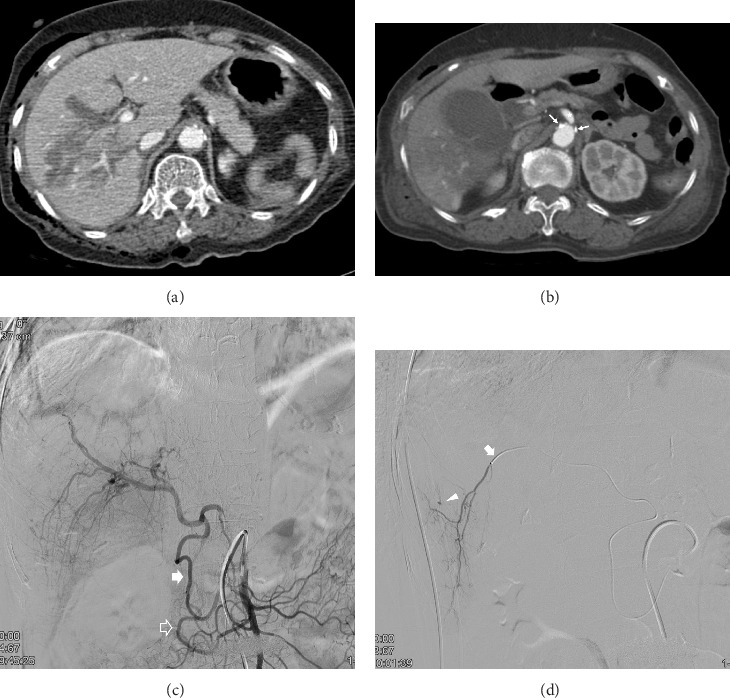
A 77-year-old female presented with blunt hepatic injury caused by a pedestrian traffic incident. (a) Contrast-enhanced (CE) axial CT during the portal phase demonstrates an irregular low-attenuation area suggestive of hepatic parenchymal laceration in the right lobe of the liver. (b) CE axial CT during the arterial phase indicates celiac axis stenosis (CAS) (represented by asterisk) with calcified plaques (arrows) at its origin. (c) Following unsuccessful attempts to cannulate the celiac axis (not depicted), superior mesenteric angiography reveals retrograde contrast material filling of the hepatic artery via the anterior (solid arrow) and posterior (open arrow) pancreaticoduodenal arcades, which originate separately from the superior mesenteric artery. (d) The microcatheter traversed the anterior pancreaticoduodenal arcade and successfully accessed the right hepatic artery (arrow). Selective angiography of the right hepatic artery demonstrated extravasation of contrast (arrowhead), which was successfully embolized using gelatin sponge particles (not shown).
